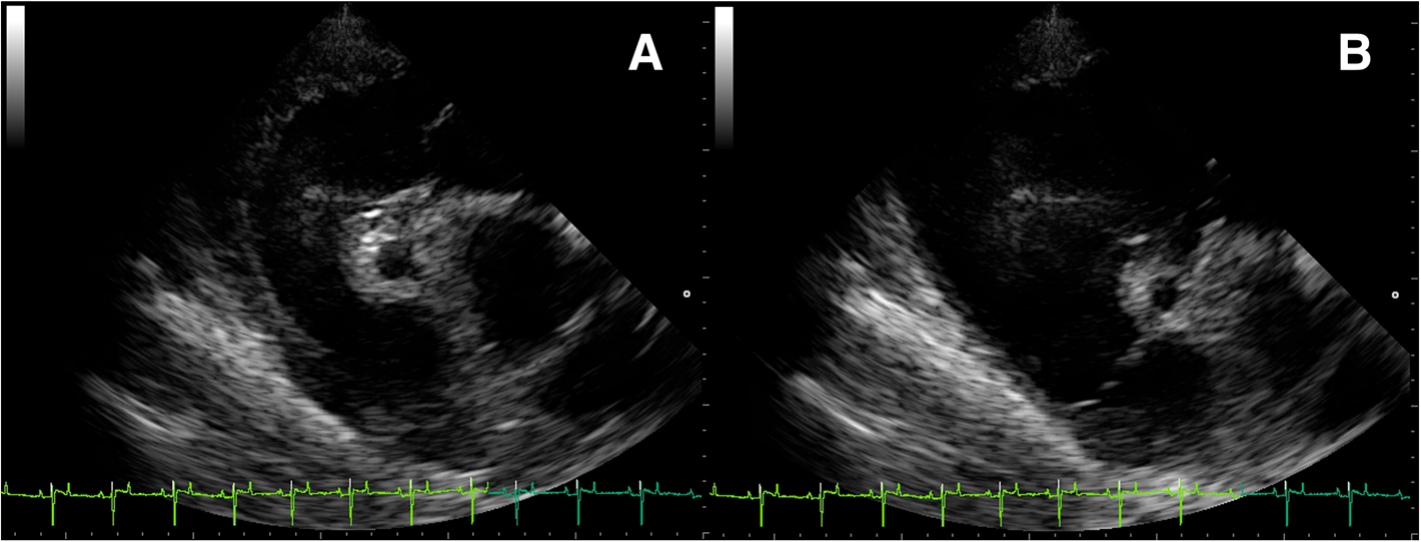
Ultrasound right parasternal long axis view of the RCA in systole (a) and diastole (b)
